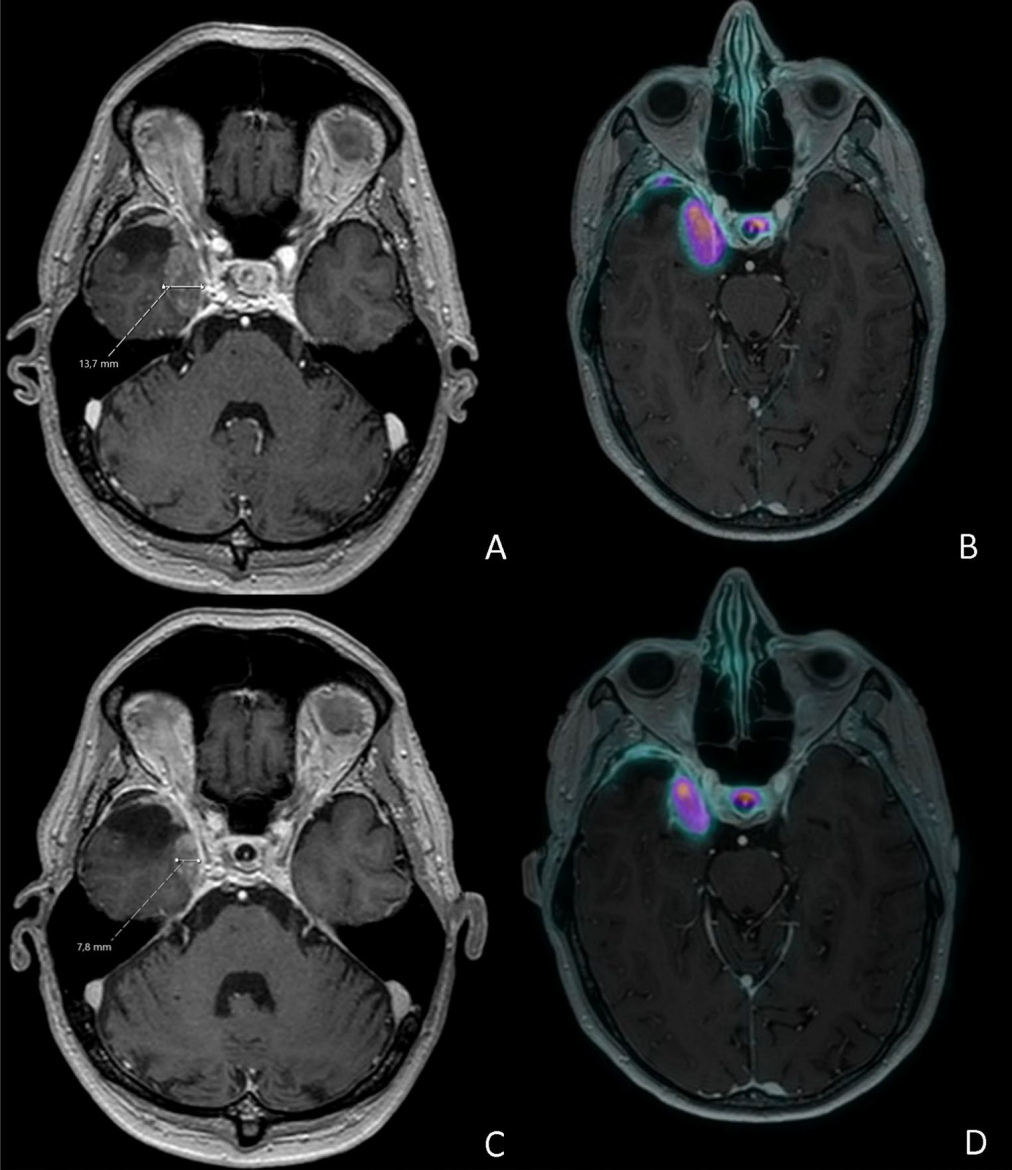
Case example (UMC-006). A Pre-treatment gadolinium-enhanced T1-weighted MRI showing a meningioma in the right sphenoid cavity with a maximum horizontal diameter of 13.8 mm. B Pre-treatment [⁶⁸Ga]Ga-DOTATOC PET/CT overlaid on MRI demonstrating high somatostatin receptor expression in the lesion. C Post-treatment gadolinium-enhanced T1-weighted MRI showing an 83% volume reduction, consistent with a partial response. D Post-treatment [⁶⁸Ga]Ga-DOTATOC PET/CT overlaid on MRI demonstrating decreased somatostatin receptor expression
